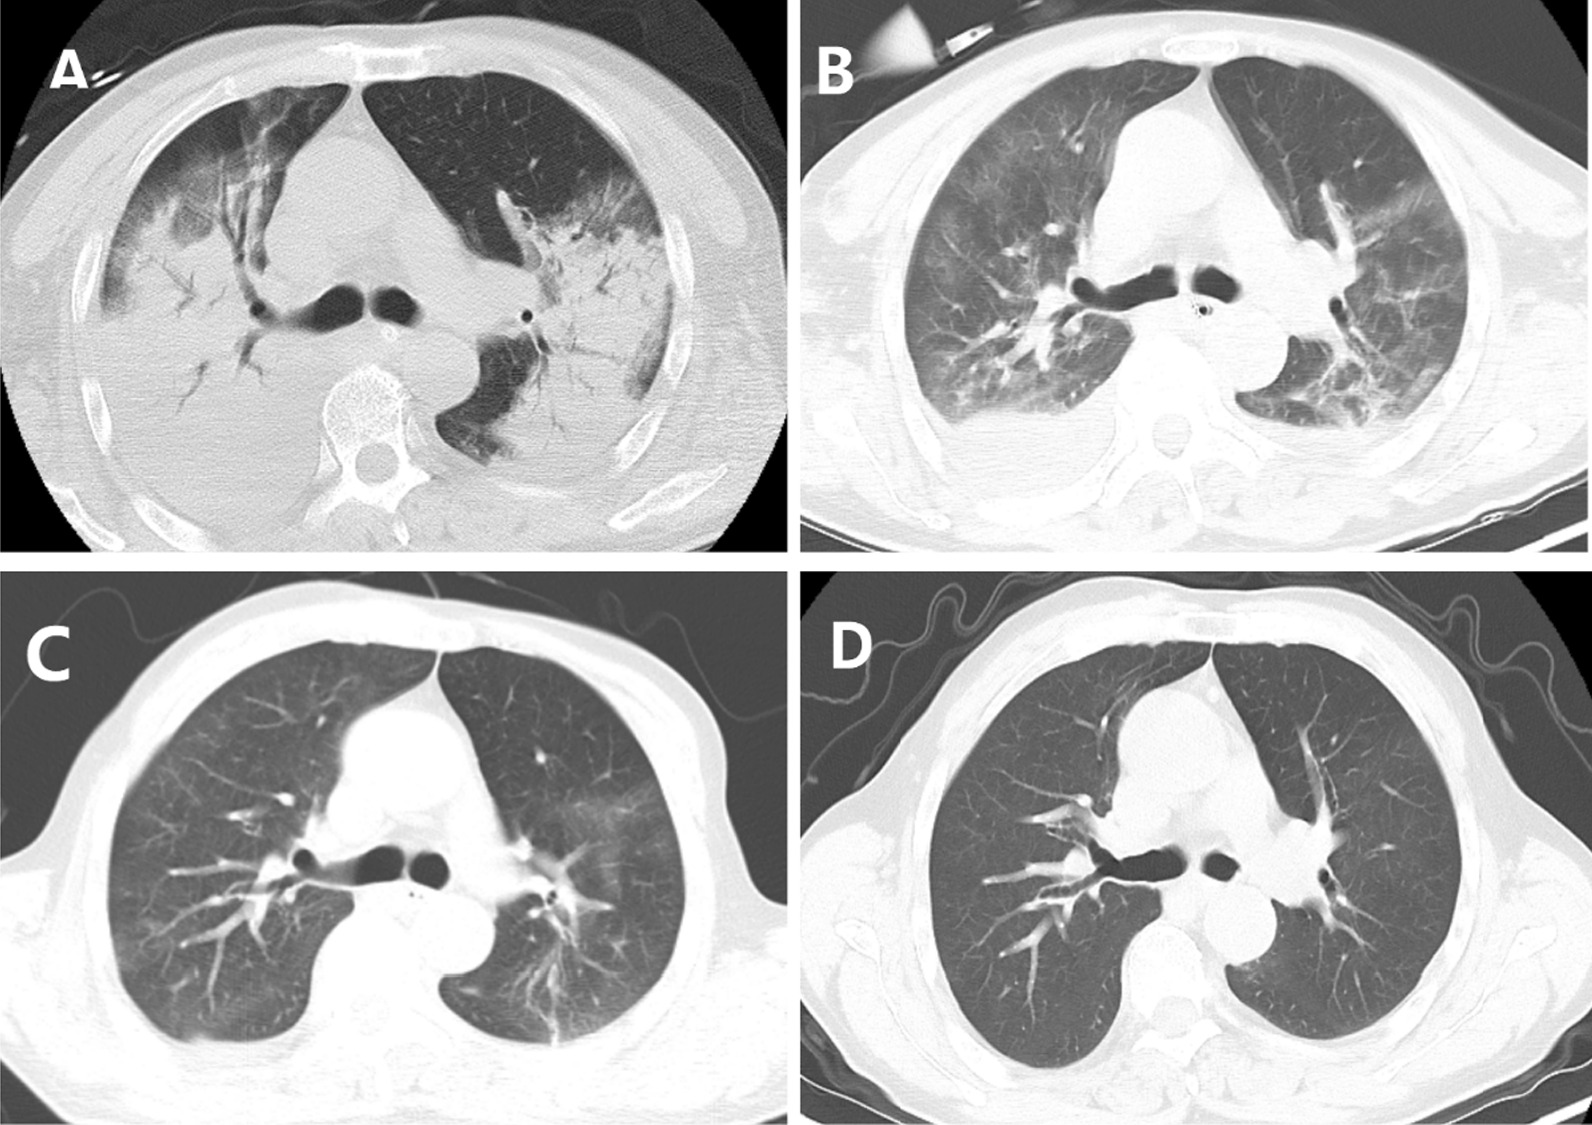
Chest computed tomography (CT) scans of a 69-year-old man with severe *psittacosis* pneumonia. The initial CT scan (6 days after onset) shows diffuse consolidation of both lungs with bronchial inflation sign (A). CT scan (14 days after the onset) shows that the consolidation gradually decreased following targeted treatment, however it showed bilateral pleural effusion (B). On follow-up (21 days after the onset), the consolidation and pleural effusion gradually decreased following treatment (C), and it completely disappeared at 66 days after onset (D)
